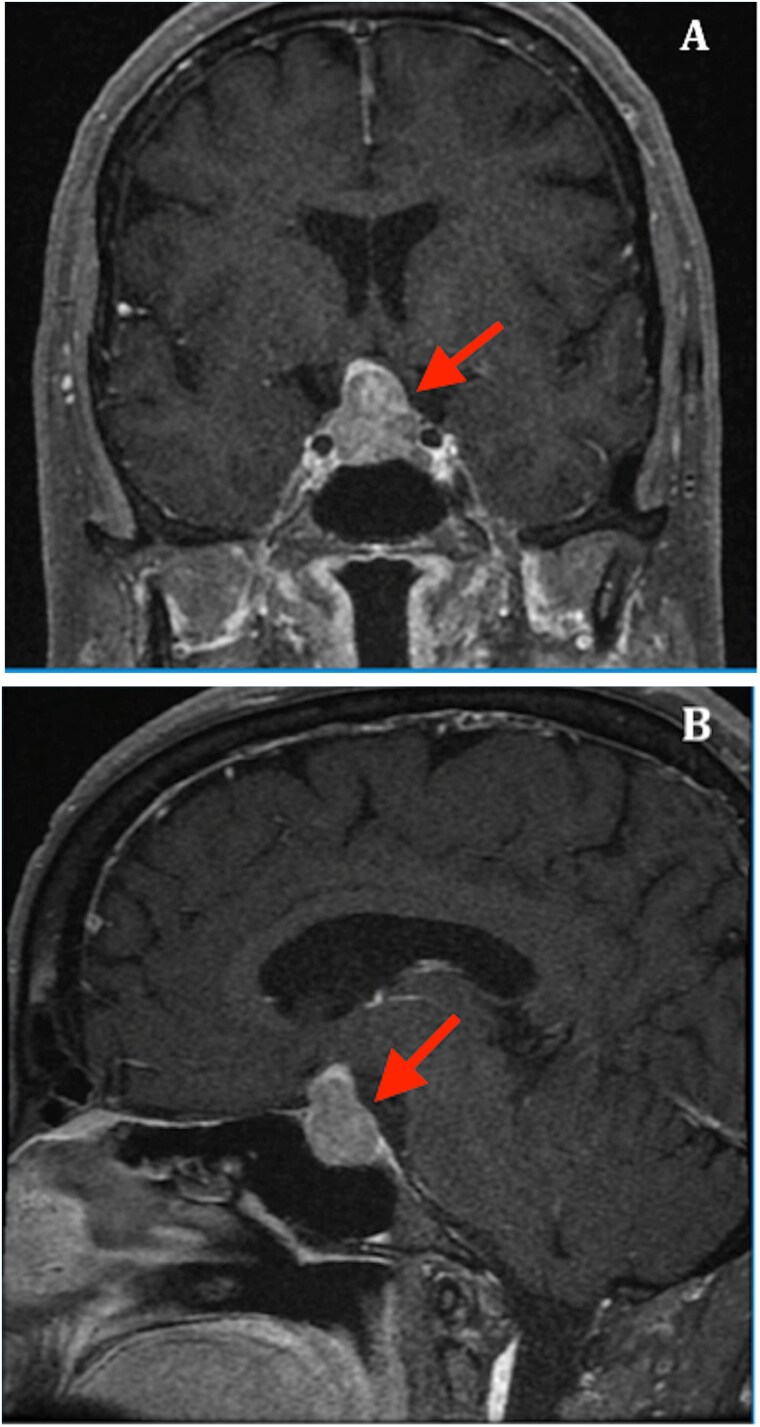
T1-weighted magnetic resonance imaging (MRI) with gadolinium contrast: (A) coronal and (B) sagittal views. The images revealed a pituitary macroadenoma measuring 22 × 18 × 18 mm, exerting superior compression of the pituitary stalk and the optic chiasm.
